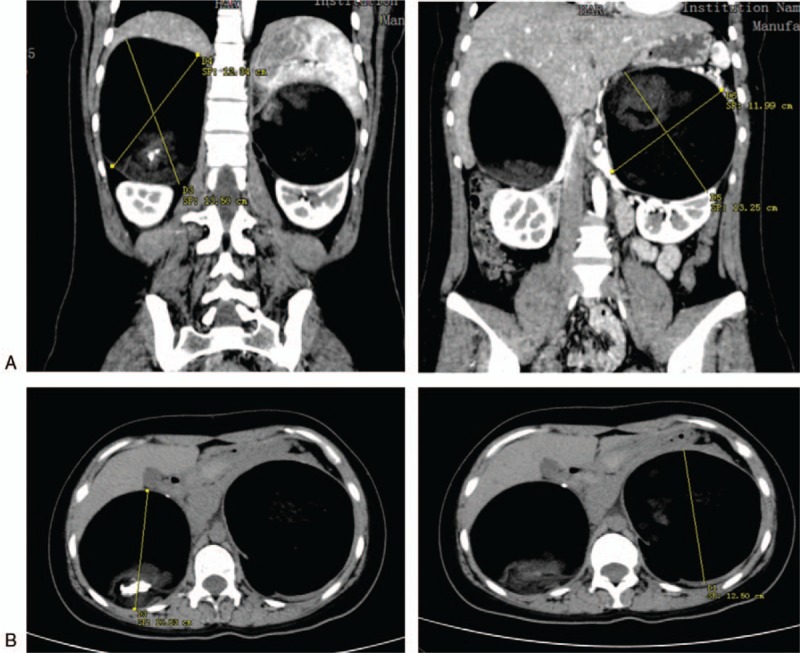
CT images of bilateral cystic teratoma: coronal view (A) and cross-sectional view (B).
